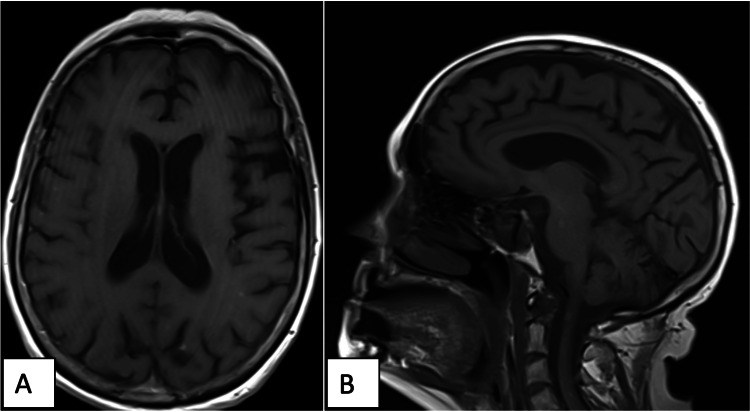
Brain MRI at 11 months after HS-WBRT showing significantly decreased size and number of metastases A) Axial and B) sagittal views. HS-WBRT: Hippocampal-sparing whole brain radiotherapy.
